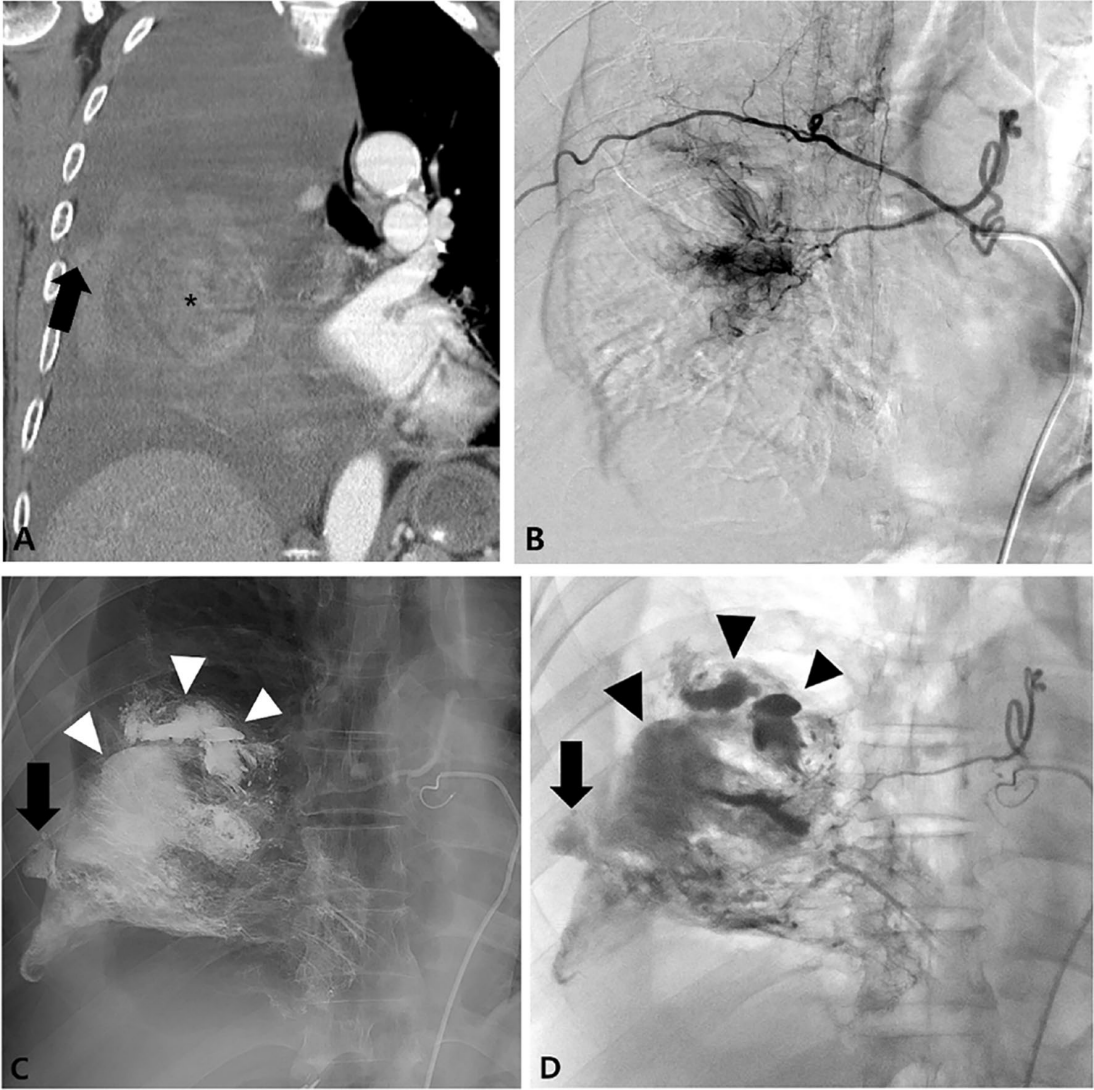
An 80-year-old man with pulmonary metastasis from HCC who presented with a trivial amount of hemoptysis and a large amount of right hemothorax. (A) Coronal reconstructed CT image before embolization shows a large enhancing mass (black asterisk) with suspicious contrast media extravasation (black arrow) in the right lower lobe and a large amount of right hemothorax. (B)Selective right bronchointercostal trunk arteriography using a 5-F catheter shows a large hypervascular tumor blush fed by the right bronchial artery in the right lower lobe. (C–D) Embolization of the right bronchial artery was successfully performed using lipiodol, gelfoam, and PVA particles, and this patient showed complete resolution of hemoptysis after bronchial artery embolization. Note the extravasation (black arrow) of lipiodol into the pleural cavity and a vascular lake phenomenon (arrowheads) resulting from intratumoral bleeding.
